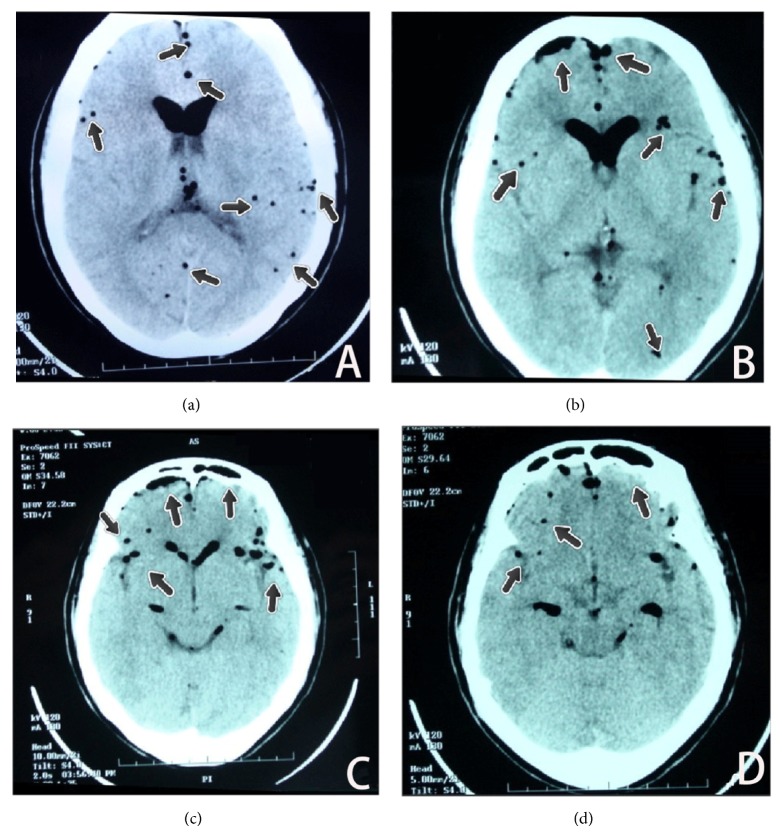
CT of the cerebral immediately after the patient evolved into light coma demonstrating multiple hypointensity spots in cerebral cortex (a), encephalocoele, right frontal lobes (b), right frontal lobes, left anterior temporal lobe (c), and anterior encephalocoele (d).
